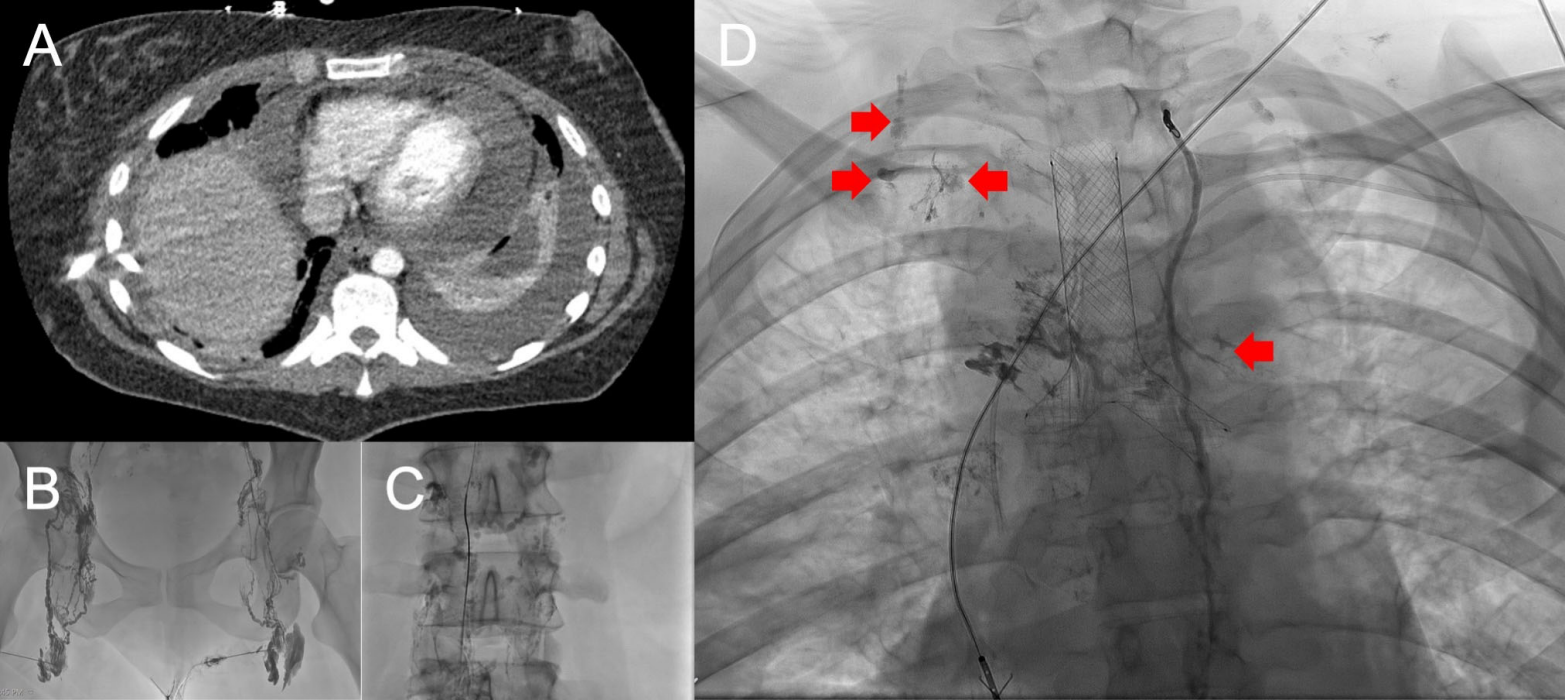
Pre-procedural images of Patient 2 show contrast-enhanced axial CT reformat (A) demonstrating large pericardial and moderate left pleural effusion. Intraprocedural AP fluoroscopic images demonstrating difficult groin nodal access (B) with eventual microwire and microcatheter crossing the cisterna chylii (C) and lymphangiogram demonstrating lipiodol extravasation in the region overlying the right upper and left upper hemithorax (D).
